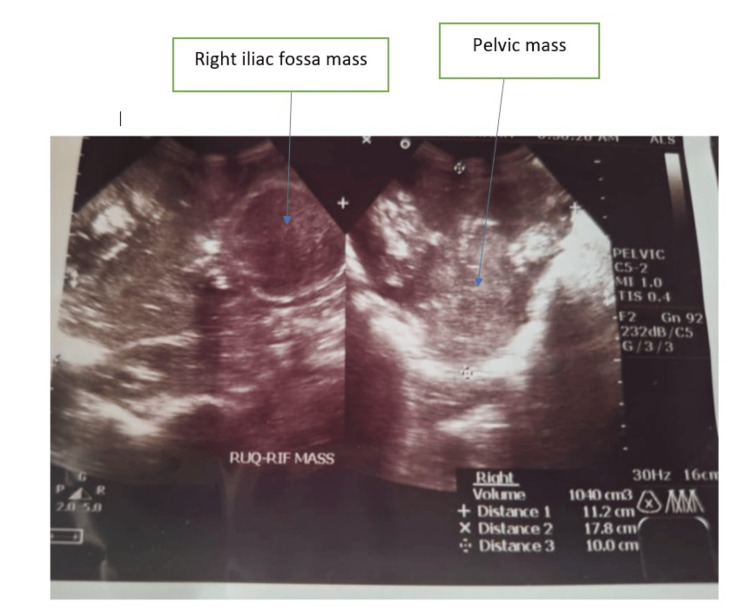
Case two abdominal ultrasound scan
